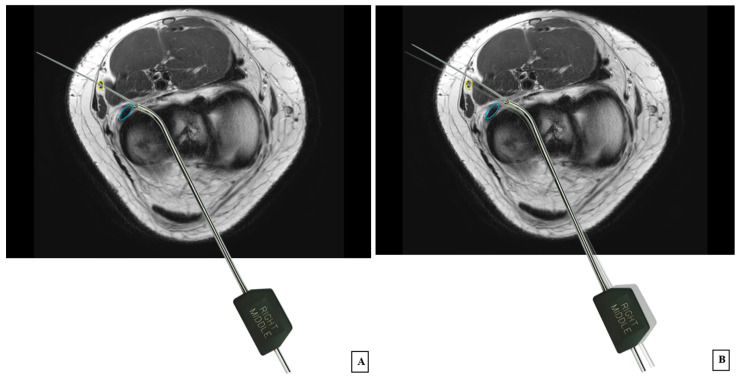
Axial MRI image of the knee showing (A) the needle passing through or touching the peroneal nerve, indicating a risk of iatrogenic peroneal nerve injury; (B) a second transparent sheet printed with the same curve as the zone-specific device was placed from the same portal to the outer border of the peroneal nerve. The “danger zone” was defined as the area between the two zone-specific devices at the level of the meniscocapsular junction. (Blue circle: popliteus tendon; yellow circle: peroneal nerve).
